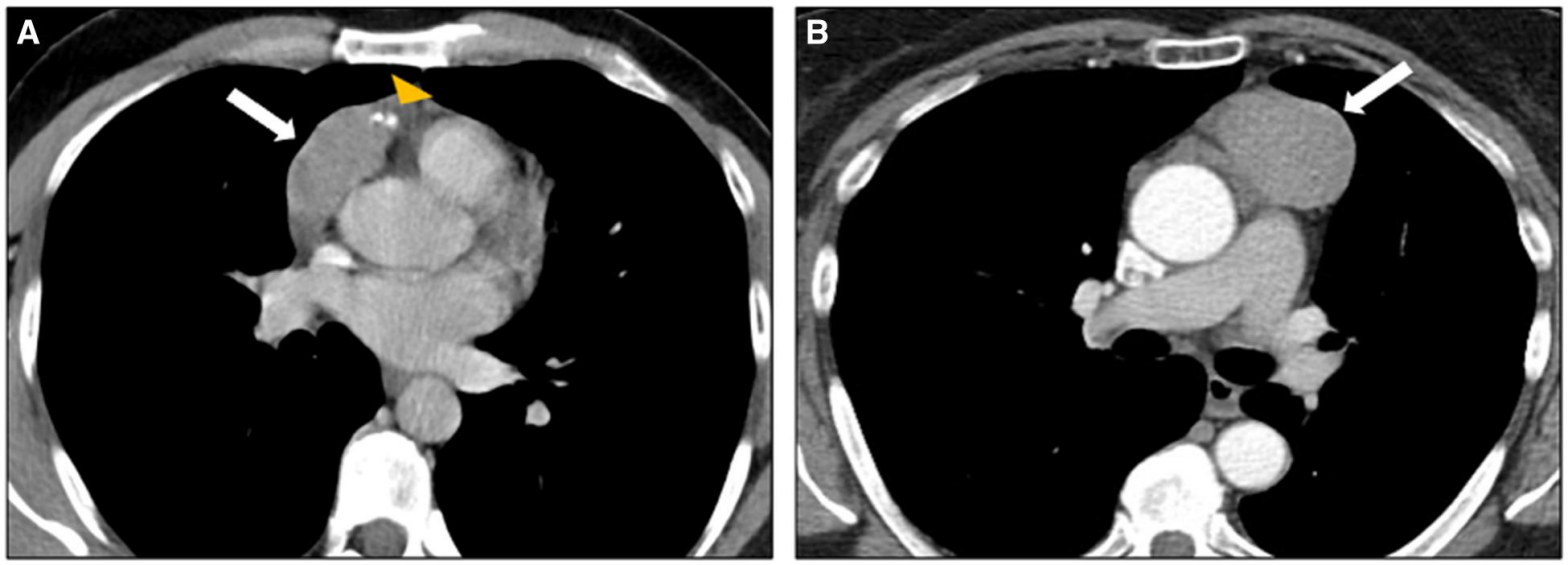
Thymoma. (A, B) Contrast-enhanced axial CT images show soft-tissue enhancing lesions (arrow) of the prevascular mediastinum, with punctate peripheral calcifications (A, yellow arrowhead). Biopsy revealed in both cases a thymoma (B2-B3, stage I Masaoka-Koga).
